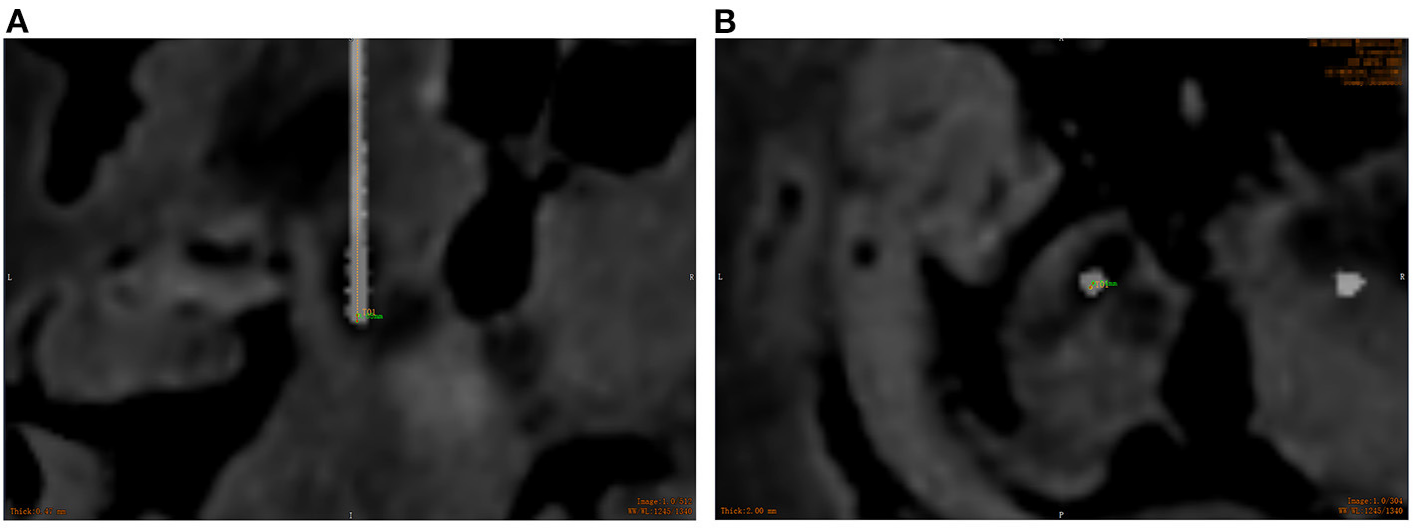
Overlay of the preoperative MR images with target trajectories and postoperative CT images to assess the electrode placement accuracy. (A) The radial error measured using the ruler tool; (B) the axial error measured using the ruler tool. (Orange represents the actual electrode position, and green represents the axial and radial error between the actual electrode and the preoperative plan).
